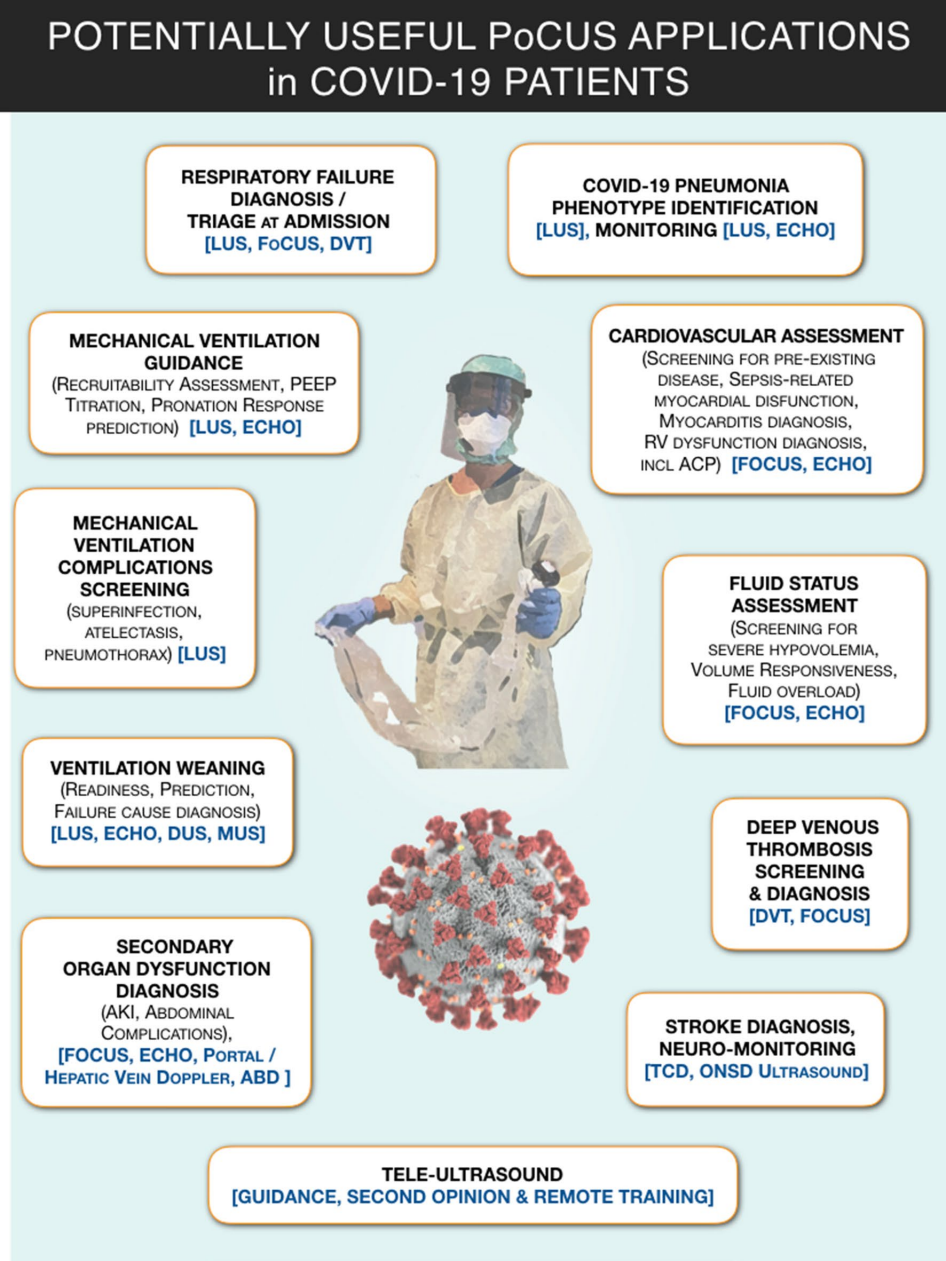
Graphical synopsis of potentially useful applications of point-of-care ultrasound (PoCUS) in COVID-19 patients. ABD, abdominal ultrasound; ACP, acute cor pulmonale; AKI, acute kidney injury; DUS, diaphragmatic ultrasound; DVT, ultrasound for deep venous thrombosis screening; ECHO, echocardiography; FoCUS, focused cardiac ultrasound; LUS, lung ultrasound; MUS, parasternal intercostal muscles ultrasound; ONSD, optic nerve sheath diameter; PEEP, positive end expiratory pressure; PoCUS, point-of-care ultrasound; TCD, transcranial Doppler; VASC, ultrasound for venous and arterial access
